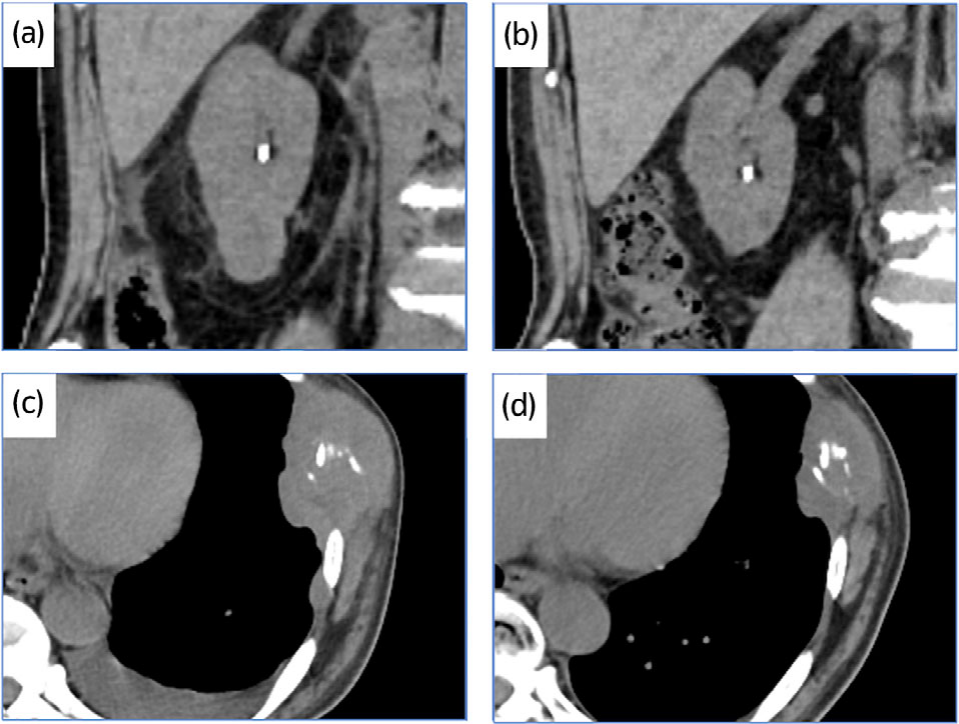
CT images in case 1. There is a right renal mass on initial diagnosis (a) and after 10 cycles of pembrolizumab and axitinib therapy (b). There is a metastatic bone lesion on the seventh rib on initial diagnosis (c) and after 10 cycles (d).
